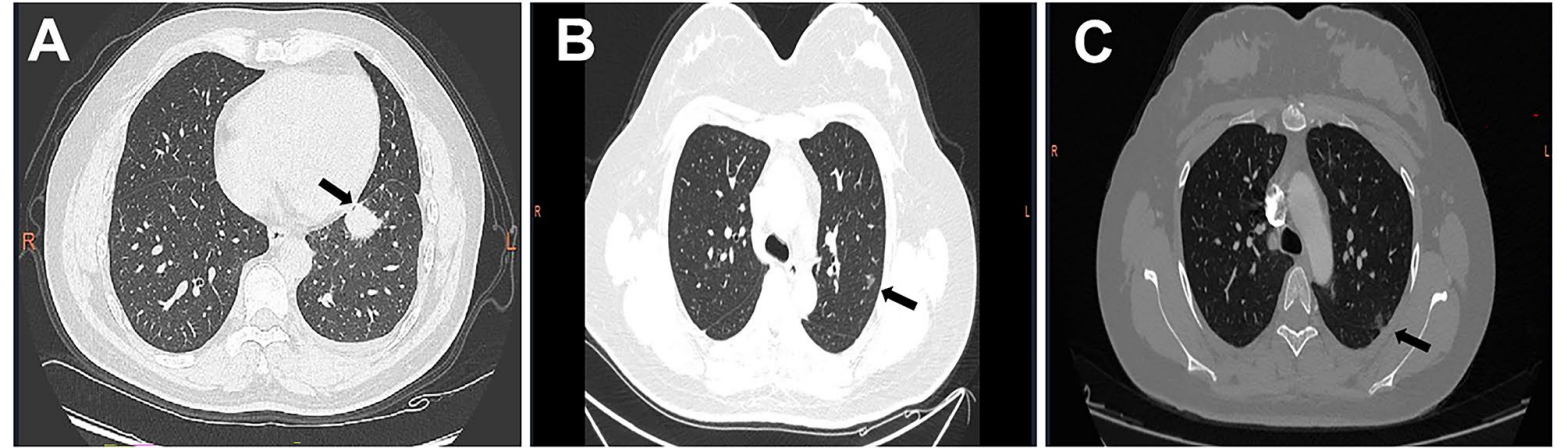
CT scans of the proband and his two daughters. A Adenocarcinoma(arrow) of the left upper lobe of the proband(II-3). B Double-lung ground-glass nodules (arrows) of the proband’s eldest daughter(III-2). C Double-lung ground glass nodules (arrows) of the proband’s youngest daughter(III-3)
